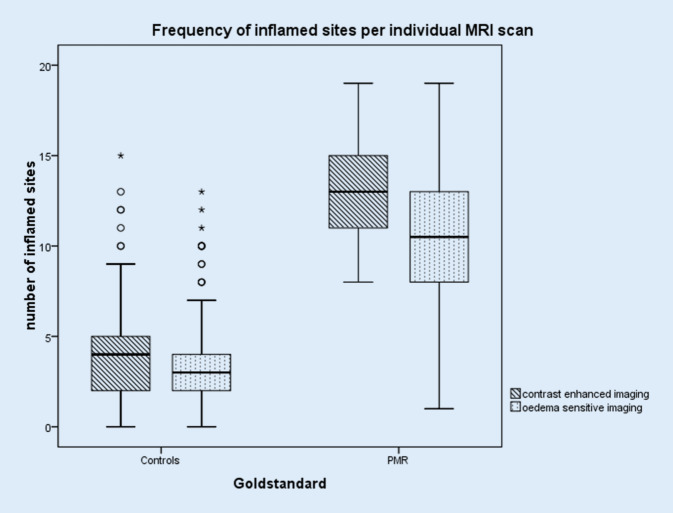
Frequency of inflamed sites per individual magnetic resonance imaging (MRI) scan in controls and polymyalgia rheumatica (PMR) cases as observed by contrast-enhanced versus oedema-sensitive imaging
